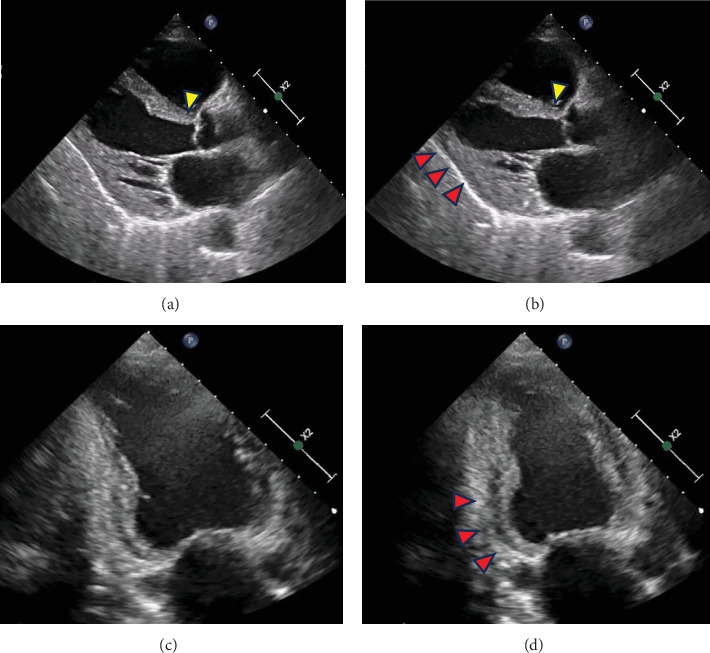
Echocardiography conducted at the time of transfer to our hospital. The echocardiography reveals thinning of the basal septal wall thickness (yellow arrowheads) and severe hypokinesis in the mid-posterior wall (red arrowhead) at (a) end-diastole and (b) end-systole and severe hypokinesis in the basal inferior wall (red arrowhead) at (c) end-diastole and (d) end-systole.
